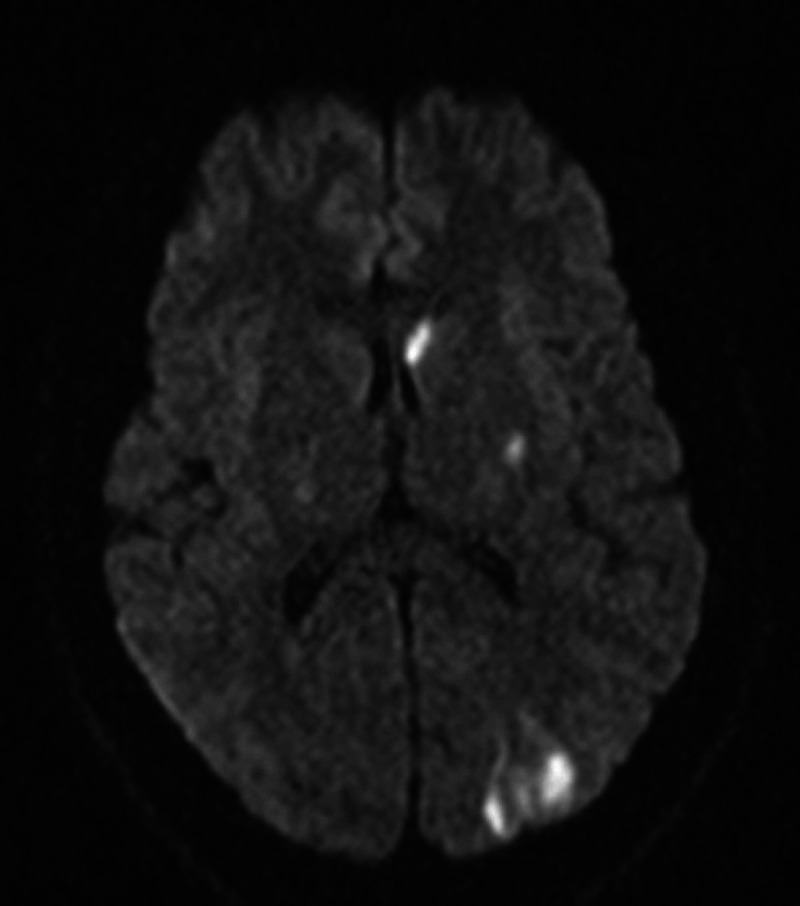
Admission MRI showing multiple hyperintensities suggestive of acute embolic stroke along left middle cerebral artery (MCA) territory
